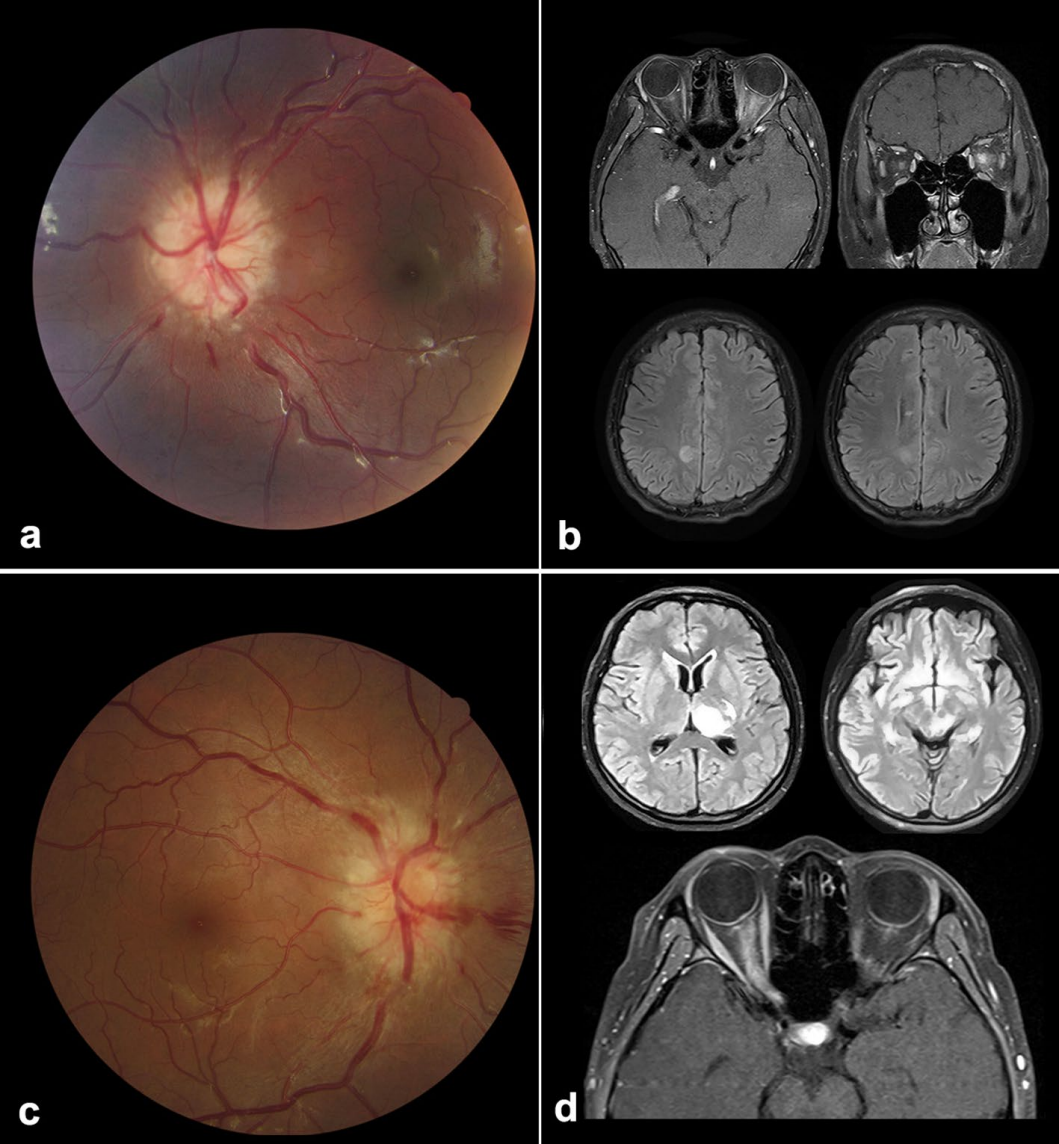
Patients who had a positive result for myelin oligodendrocyte glycoprotein antibodies presenting with brain lesion without diagnosis. (a, b) Images of a 12-year-old boy presenting with acute visual loss in the left eye for 10 days. One month ago, he experienced sudden loss of consciousness and a seizure. Fundoscopy (a) shows obvious edema of the optic disc, and brain and orbit magnetic resonance imaging (MRI) (b) shows enlarged and enhanced left optic nerve with brain lesions, indicating acute disseminated encephalomyelitis-like demyelinating disease, which was the probable cause for the seizure. (c, d) Images of a 30-year-old woman presenting with acute visual decrease in the right eye for 2 weeks. One month ago, she complained of weakness in her right body, while brain MRI showed a midbrain lesion, which recovered spontaneously. Fundoscopy (c) shows a severe swelling of the optic disc, and orbit MRI (d) shows extensive enhancement of the nerve.
